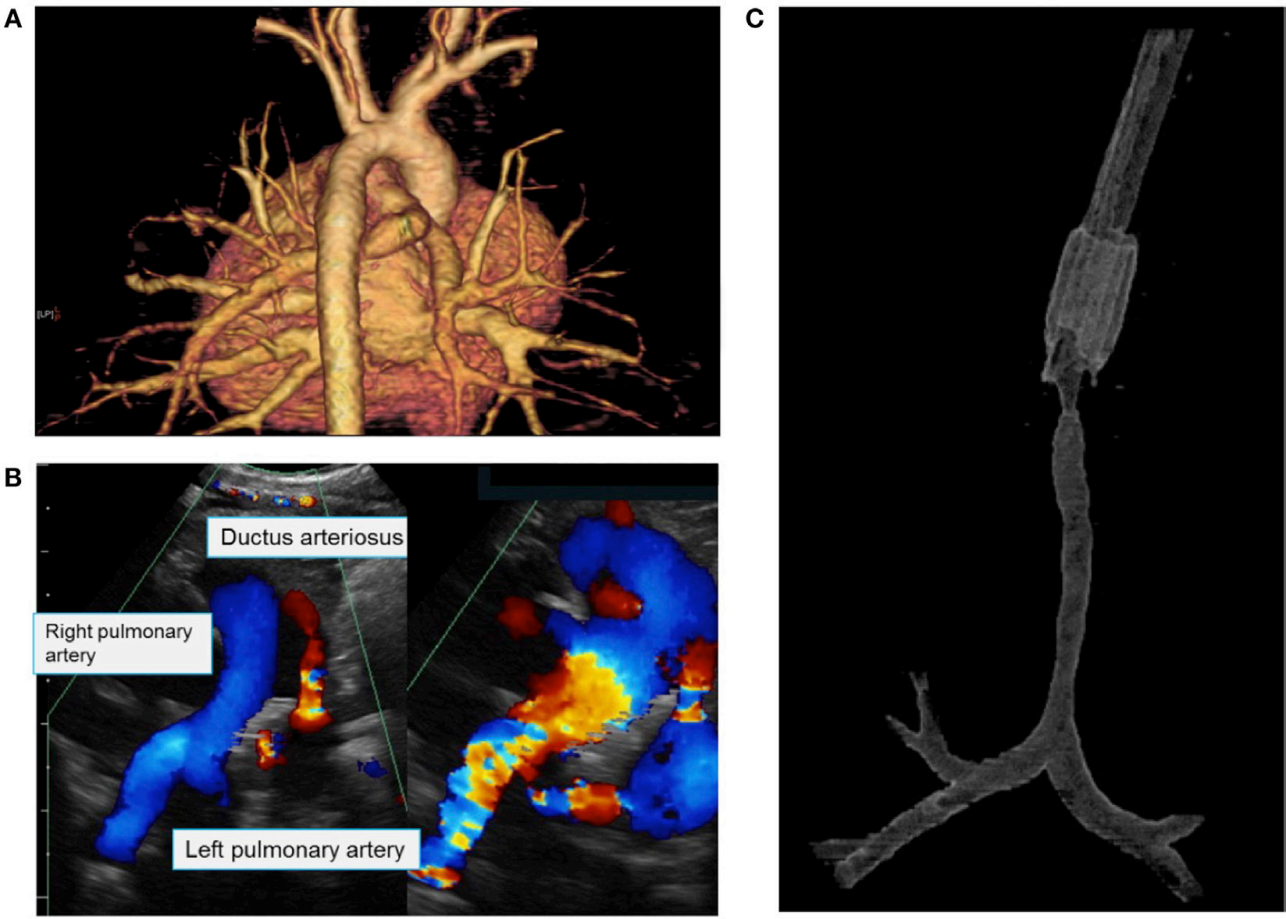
(A) 3D reconstruction of the great vessels based on a chest CT showing the diagnosis of pulmonary artery sling with aberrant origin of the left pulmonary artery (LPA) from the right pulmonary artery. (B) Echocardiography showing the main pulmonary artery, the right pulmonary artery and the LPA originating from the right pulmonary artery, as well as patent ductus arteriosus. (C) 3D reconstruction of the upper airways based on a chest CT showing long-segment tracheal stenosis beginning at the fifth tracheal ring and ending 5 mm above the carina.
